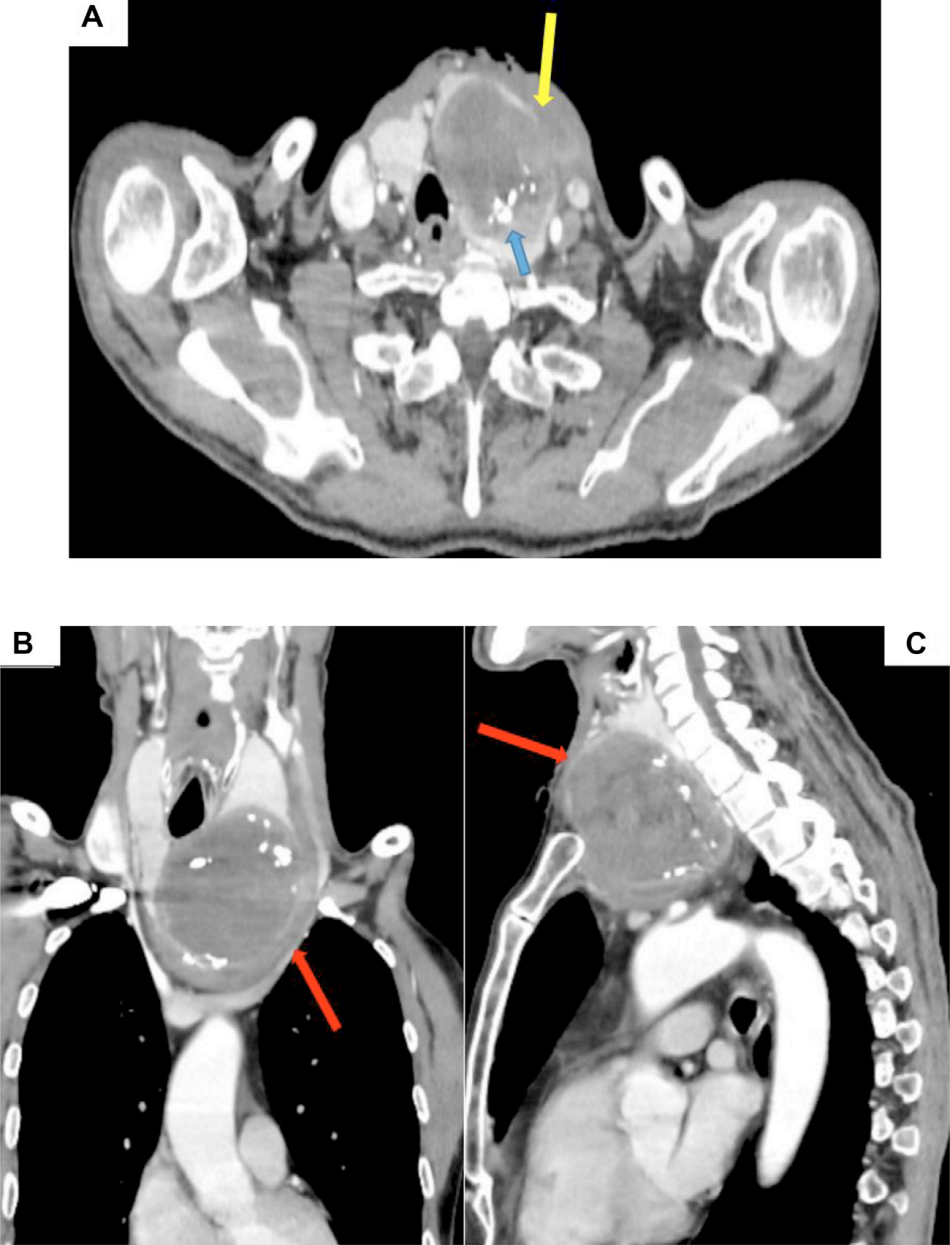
Cervicothoracic CT scan. Axial (A), sagittal (B), and coronal (C) reconstructions. showing a plunging left goiter with a large inferior polar collection (81 × 89 × 83 mm) (red arrow),containing calcifications (blue arrow). and no postcontrast enhancement, associated with a fistulous tract extending to the subcutaneous plane with soft tissue infiltration (yellow arrow), displacing the tracheoesophageal axis posteriorly, the left sternocleidomastoid muscle laterally, and extending into the anterior superior mediastinum, while exerting a mass effect on the left internal jugular vein and brachiocephalic veins, which remain patent, without direct contact with the brachiocephalic trunk or right subclavian artery.
